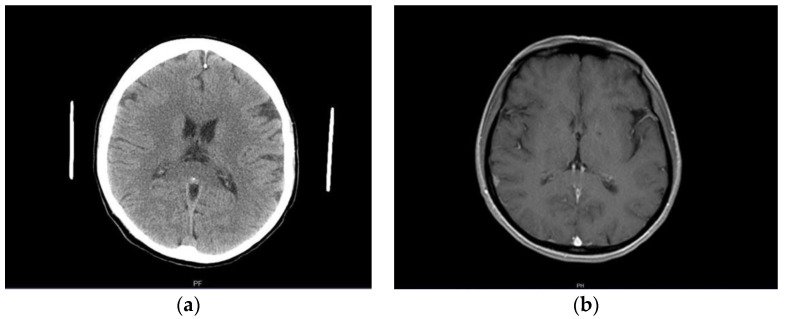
(a) Normal brain image—CT examination in native phase; (b) contrast-enhanced T1-weighted axial MR cross-section indicating a small right parietal meningioma (arrow).
